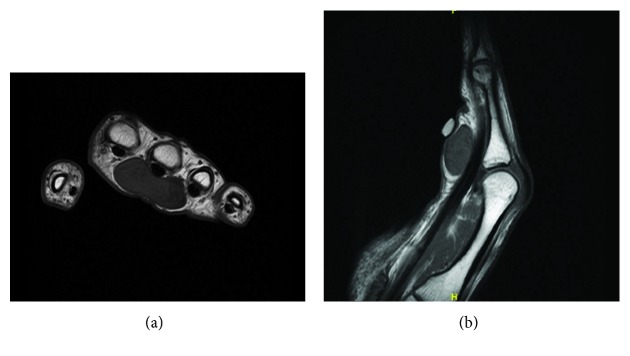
(a, b) Select preoperative T1 axial and T1 sagittal magnetic resonance images of FTS demonstrating relationship to flexor tendon and neurovascular bundle.
